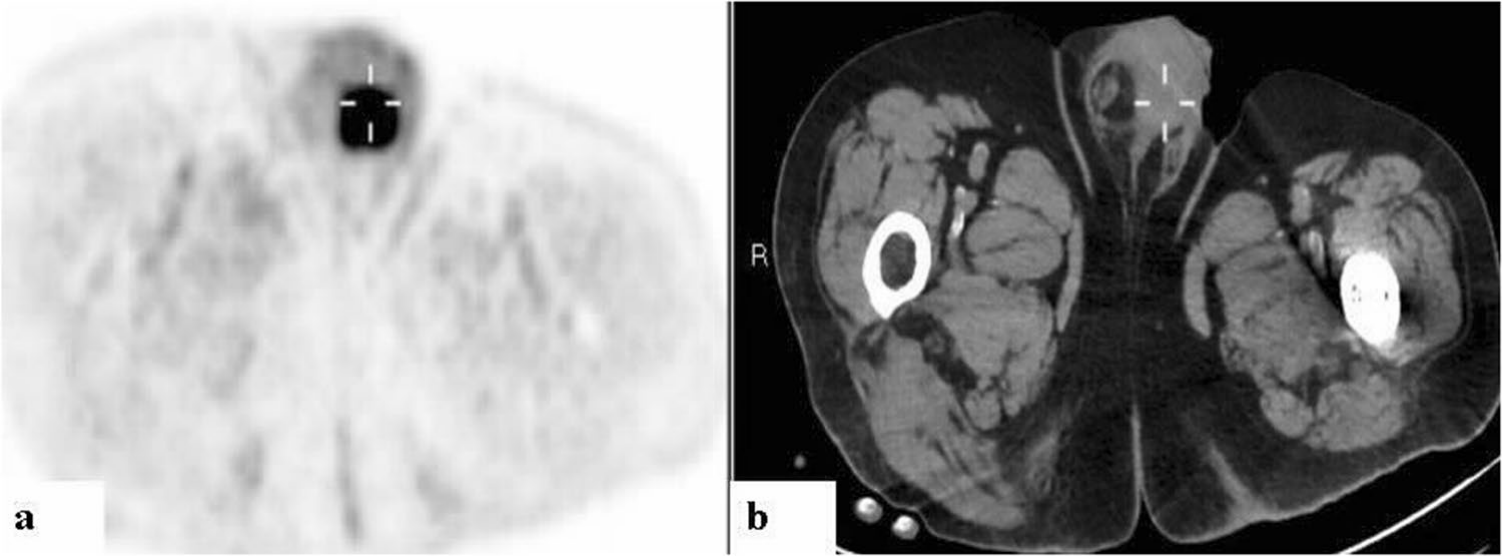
18F-FDG PET (a) and CT (b) axial slices demonstrating focally increased uptake at the root of the penis. An 80-year-old man with metastatic high-grade pelvic leiomyosarcoma (patient #23, Table 1).
